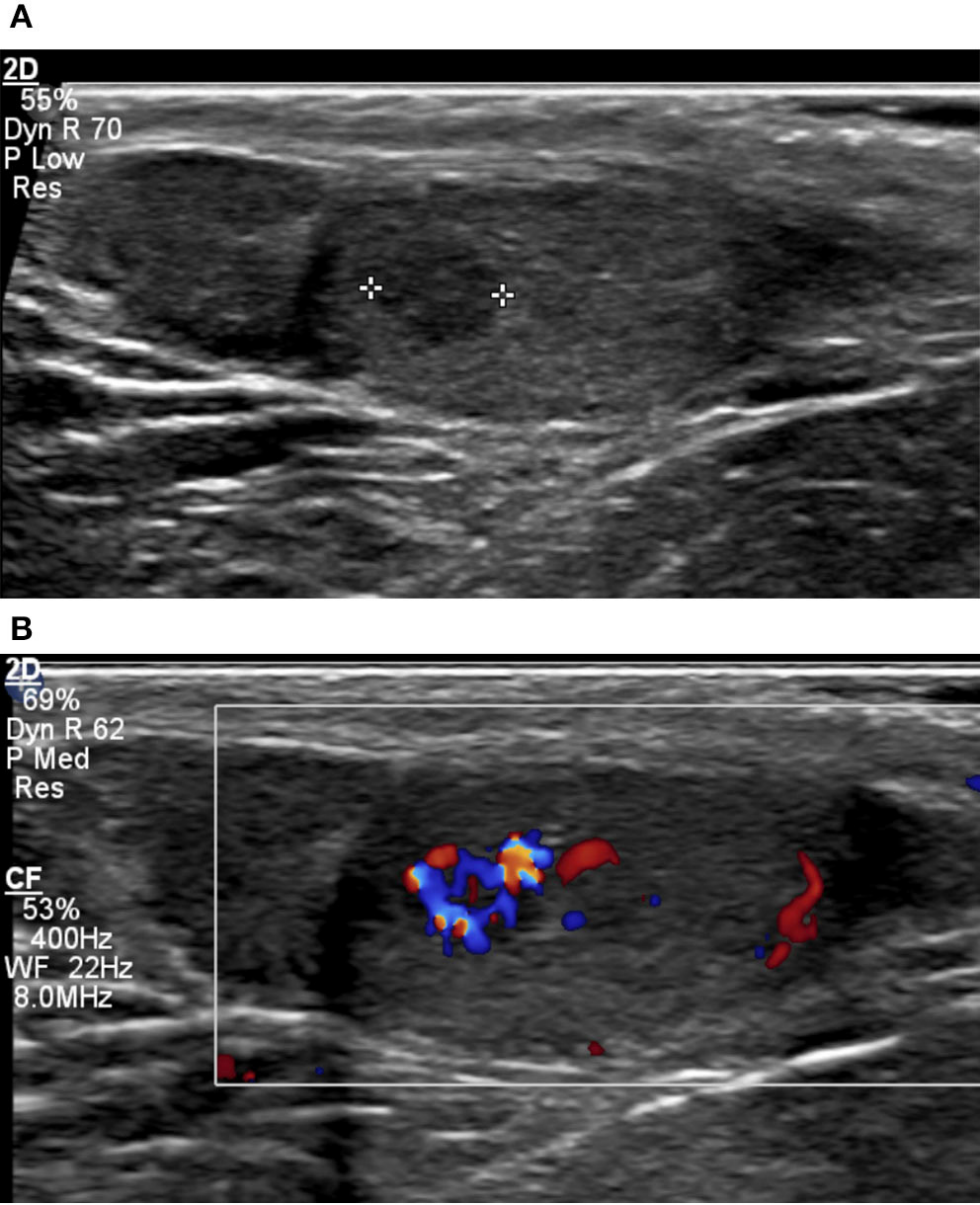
Small-volume testis (2 ml) in a 36-year-old patient with KS, characterized by coarse background echotexture (A). A 4-mm hypoechoic lesion with markedly increased vascularity on color Doppler (B) was found to correspond to nodular Leydig cell hyperplasia on surgical biopsy.
